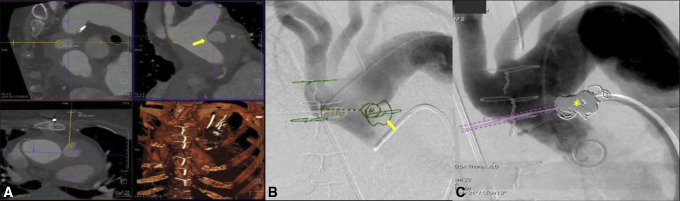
Planned virtual needle path. A, Preprocedural cardiac computed tomography angiography (CCTA) was used to map a needle trajectory into the ascending aortic pseudoaneurysm (*arrow*), avoiding vital structures. B, The CCTA map demonstrating the location of the pseudoaneurysm and other anatomical landmarks is fused with live fluoroscopy to allow needle insertion under supervision. C, Completion angiography demonstrates exclusion of the pseudoaneurysm by coil embolization (*asterisk*) deployed through a percutaneous needle (highlighted by pink tracks).
